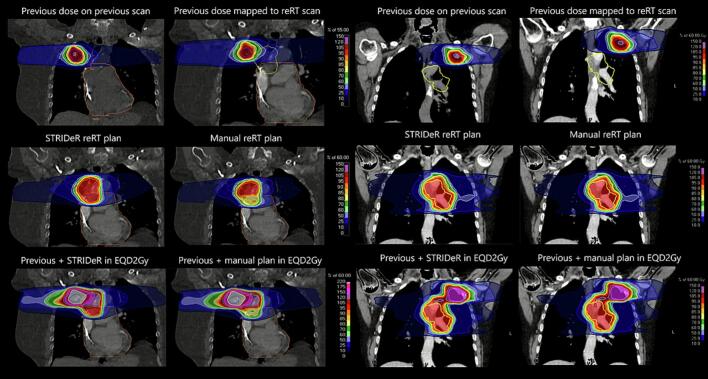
Previous and reirradiation images and treatment plans for Patient 3 (left) and Patient 1 (right). Patient 3: The previous plan delivered 55 Gy in 5 fractions to the planning target volume (PTV) (upper left, blue contour, 49.0 cm^3^). The previous dose was mapped onto the reirradiation (reRT) CT (upper right). The Support Tool for ReIrradiation Decisions guided by Radiobiology (STRIDeR) approach permits coverage of the inferior section of the reRT PTV (centre left) compared with the manual approach (centre right). Lower images show the cumulative dose in EQD2Gy for STRIDeR (left) and manual (right) plans. Orange contour: pericardium; yellow contour: reRT PTV (56.7 cm^3^). Patient 1: The previous plan delivered 60 Gy in 8 fractions to the PTV, left upper lobe (36.7 cm^3^). The second treatment was in the left lower lobe, PTV volume (275.8 cm^3^). Both planning methods produced very similar plans, demonstrating that in some cases there is little dosimetric benefit; however the STRIDeR method was considered less burdensome and more automated by the planner.
